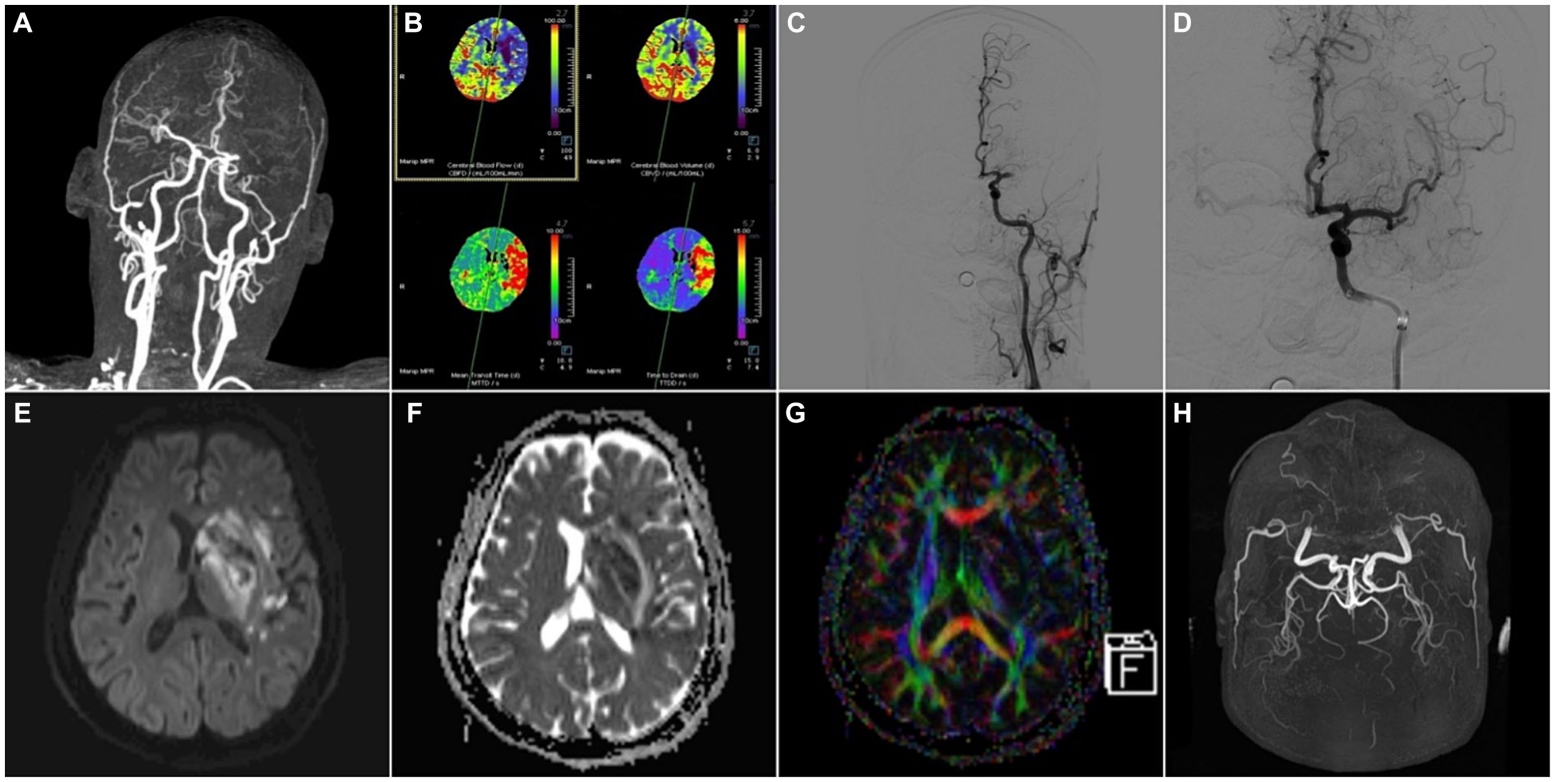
A 59-year-old male patient was admitted to the hospital with “weakness of right limb for 13 h” and was treated with arterial thrombectomy. (A) CTA-MIP: occlusion of the left middle cerebral artery; (B) PCT: the value of CBF, CBV became lower and the value of MTT, TTD became longer in the left middle cerebral artery supply area compared to the right, which meant hypoperfusion in the left middle cerebral artery supply area; (C,D) DSA: angiography suggested occlusion of the left middle cerebral artery, and after thrombectomy, re-angiography showed that the main trunk of the left middle cerebral artery was patent, with some thrombus escaping to the distal branches; (E) MRI-DWI, (F) MRI-ADC, (G) MRI-DTI-colorful FA: 3 days after thrombectomy, DWI and ADC demonstrated multiple infarct foci in the blood-supplying area of the left middle cerebral artery, and a few hemorrhagic transformed foci in the basal ganglia area, DTI showed the left nerve fiber bundles were thinner than those on the right side; (H) MRA-TOF: 4 days after thrombectomy, the left middle cerebral artery was still patent.
